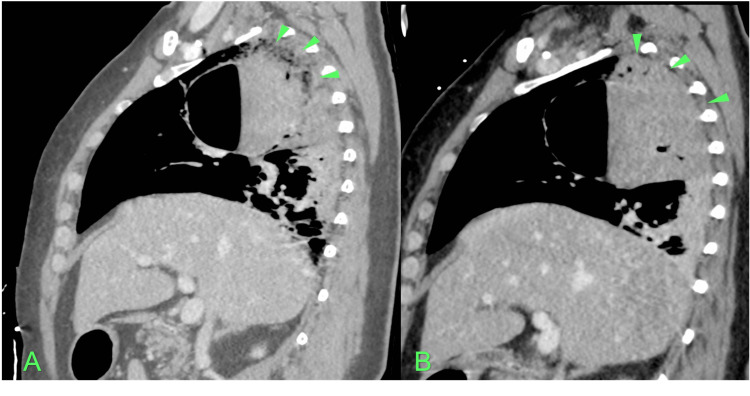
Sagittal plane of the first (A) and second (B) Thoracic CTA where’s noted an increase in the densification of the adjacent pulmonary parenchyma compatible with hematic alveolar filling (Green arrows).
